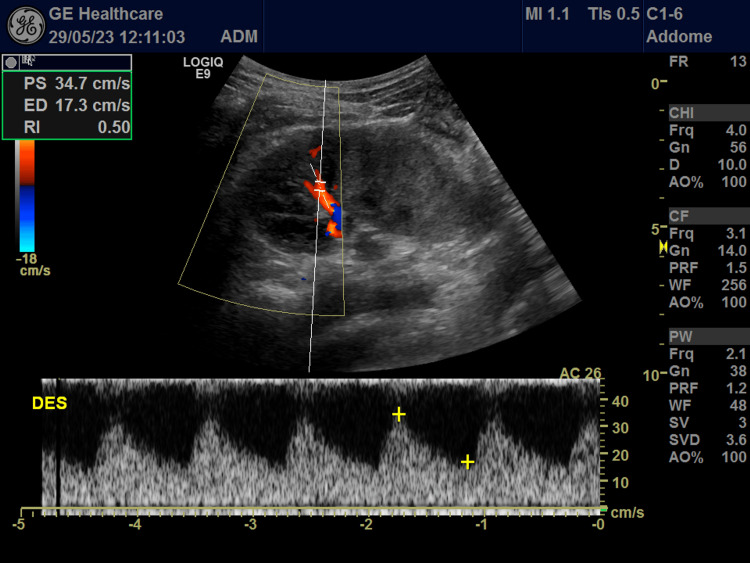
Color Doppler ultrasound of the right renal artery, flowmetric sampling at the level of the intracortical branches Flow paths with reduced resistance index.
